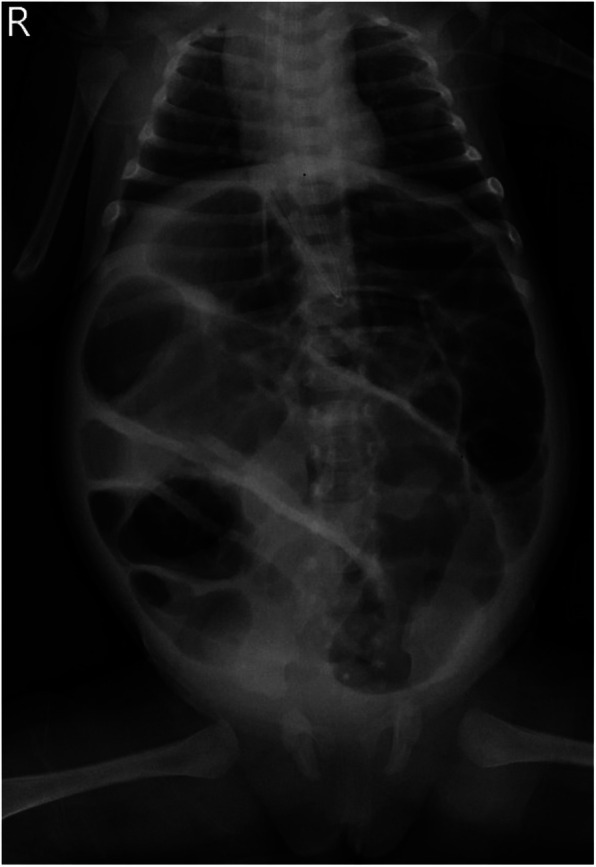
Neonatal abdominothoracic x-ray.
